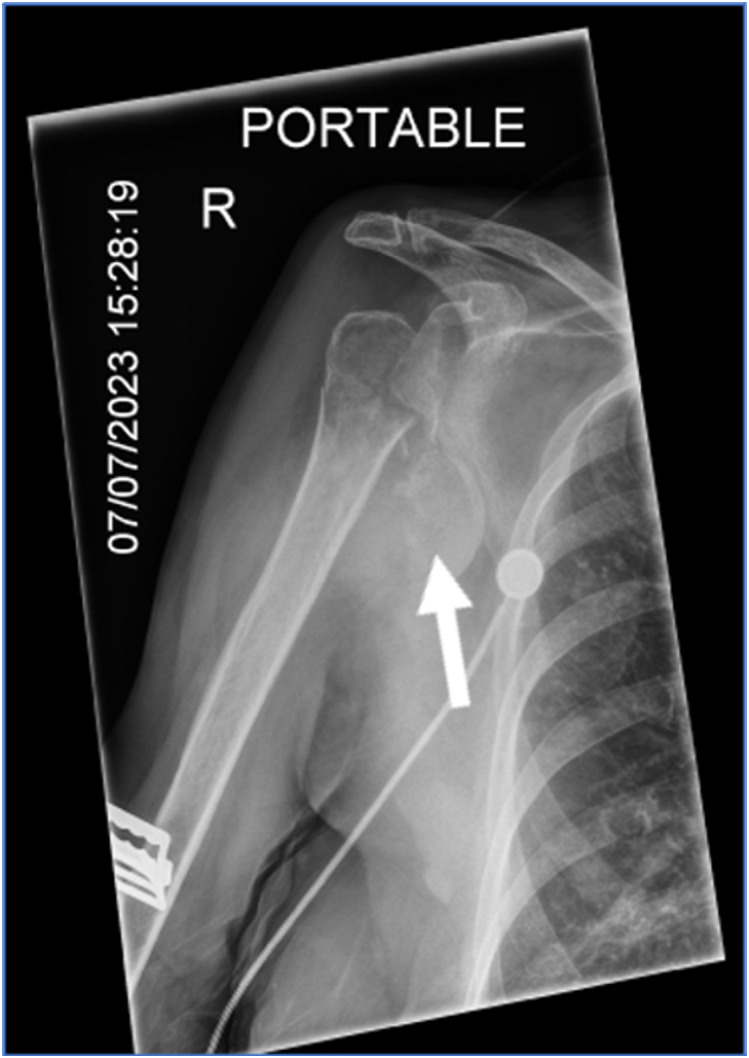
Radiograph of right shoulder immediately post-reduction showing humeral fracture (arrow).
